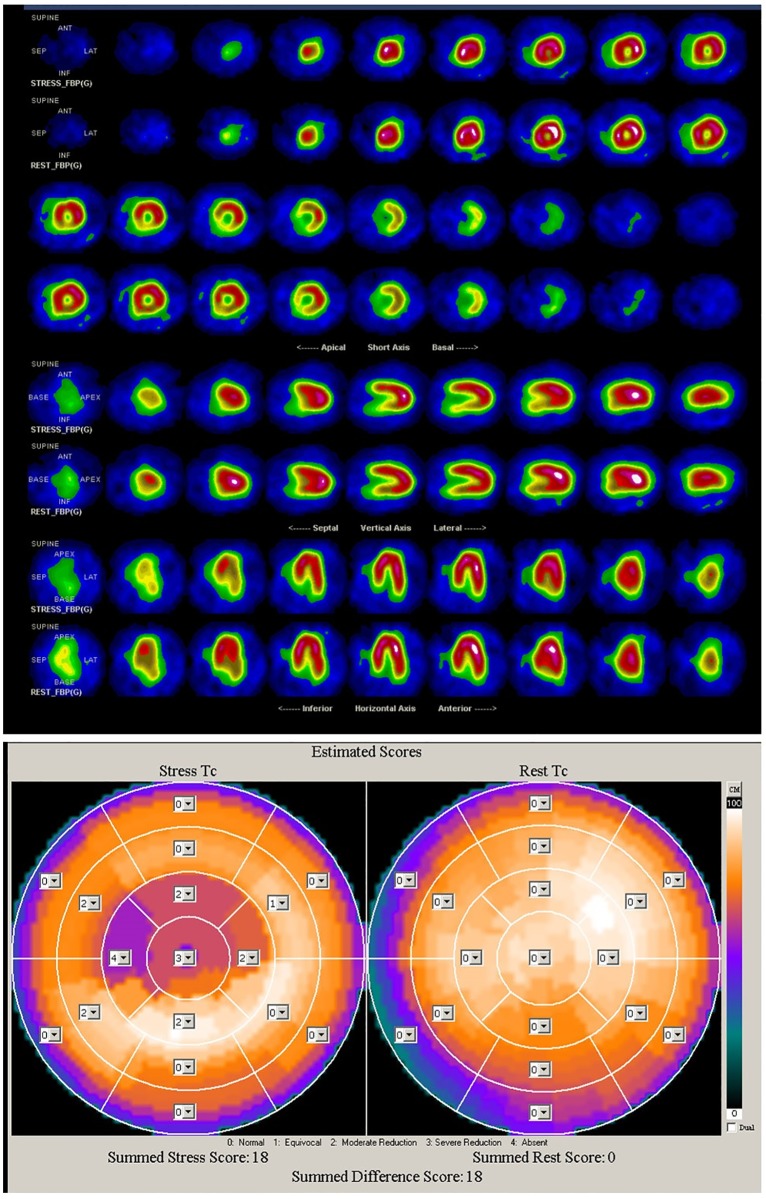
SPECT MPI results of GB0880. The rest and stress SPECT ischemia score of GB0527 are 0 and 18, respectively.
